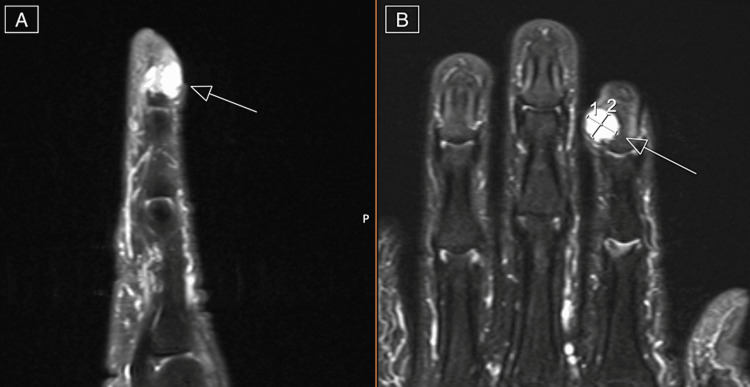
T2-weighted fat-suppressed images of MRI revealing hyperintense possible ostial lesion of the distal phalanx. (A) Sagittal view. (B) Coronal view.
